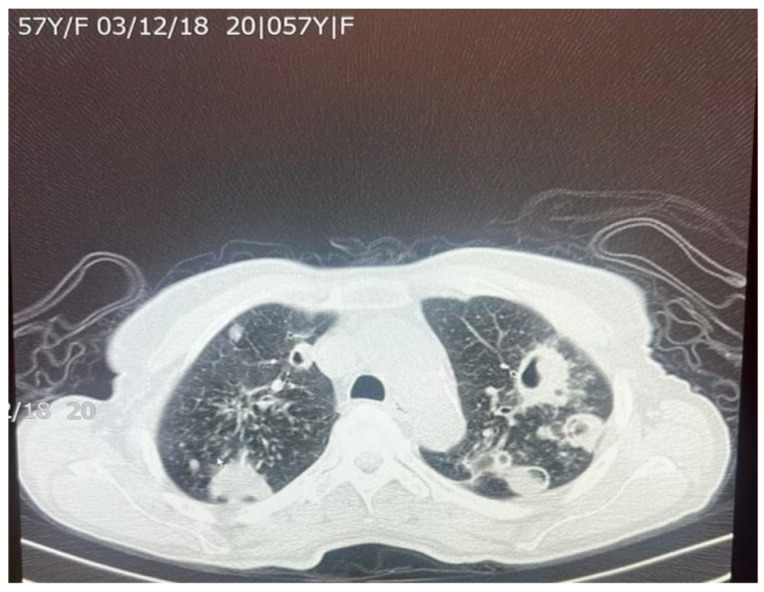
An axial chest CT scan of the same patient in Figure 5, demonstrating advanced nodular and cavitary changes in both lungs, consistent with pulmonary TB. The bilateral upper lobe cavities are clearly visible with associated nodules. The distribution of the lesions, with upper lobe involvement and cavitation, is indicative of reactivation of TB. Additionally, areas of tree-in-bud appearance suggest bronchogenic spread of the infection, which is common in TB patients.
